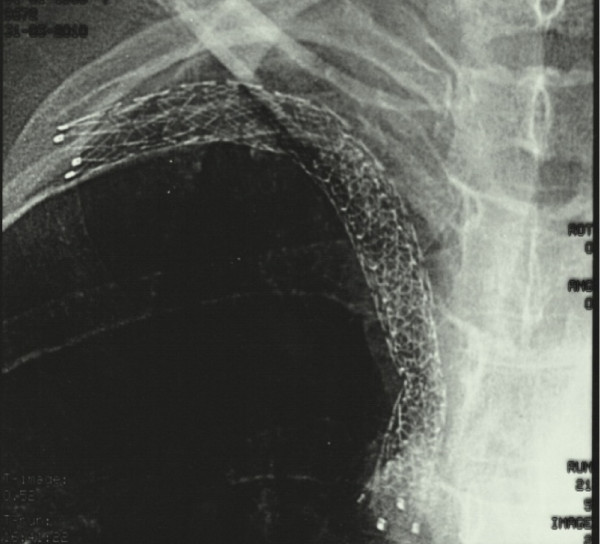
Chest radiograph showing the stent placed in the right subclavicular vein and superior vena cava through thrombosis.
